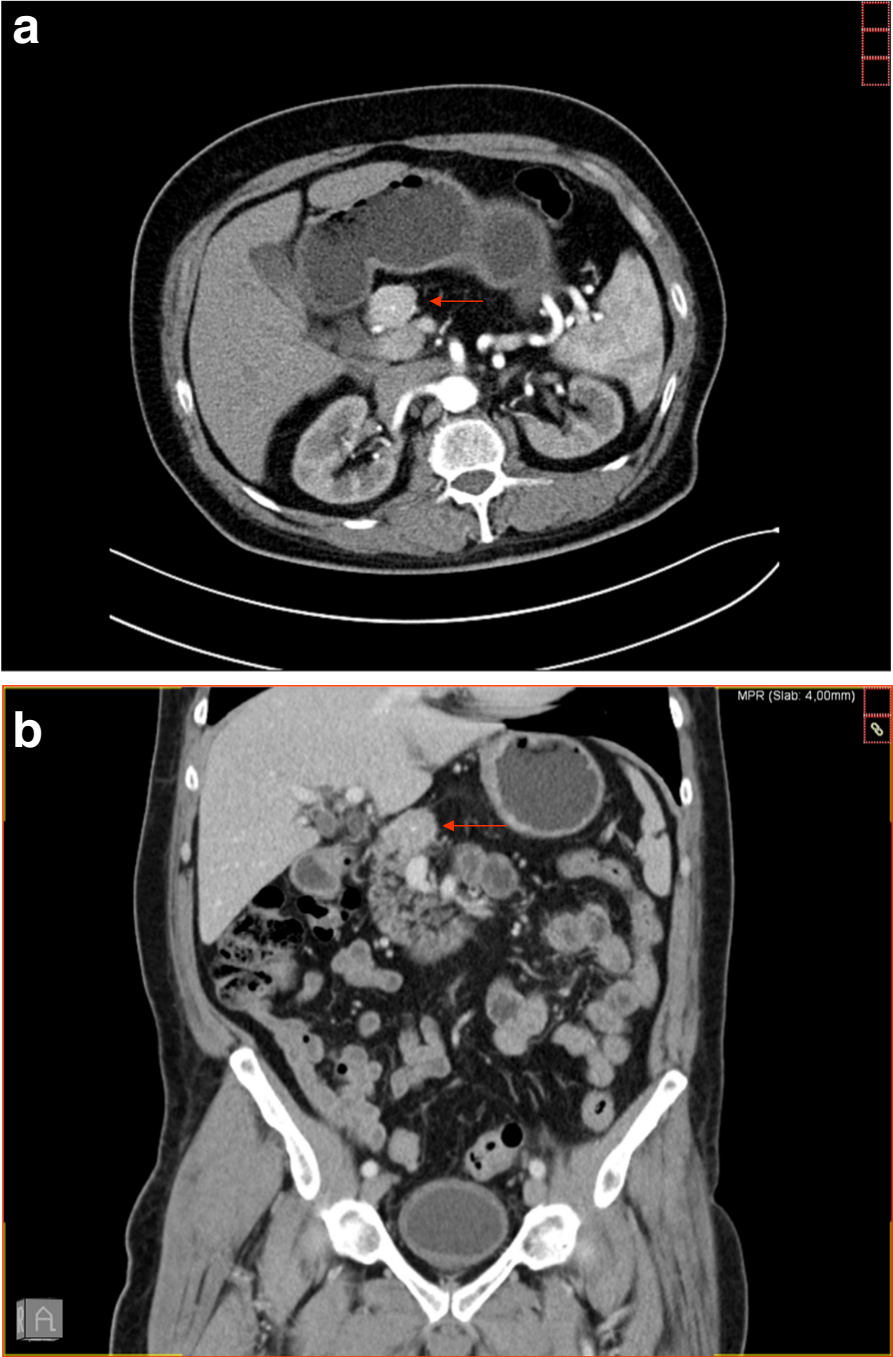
Contrast-enhanced computed tomography scan with axial image in arterial phase (a) and coronal reconstructed image in venous phase (b) showing a round hypervascular mass, measuring approximately 3 cm at the neck of the pancreas with absence of the body and tail of the pancreas. The *arrows* show the lesion in the pancreas
